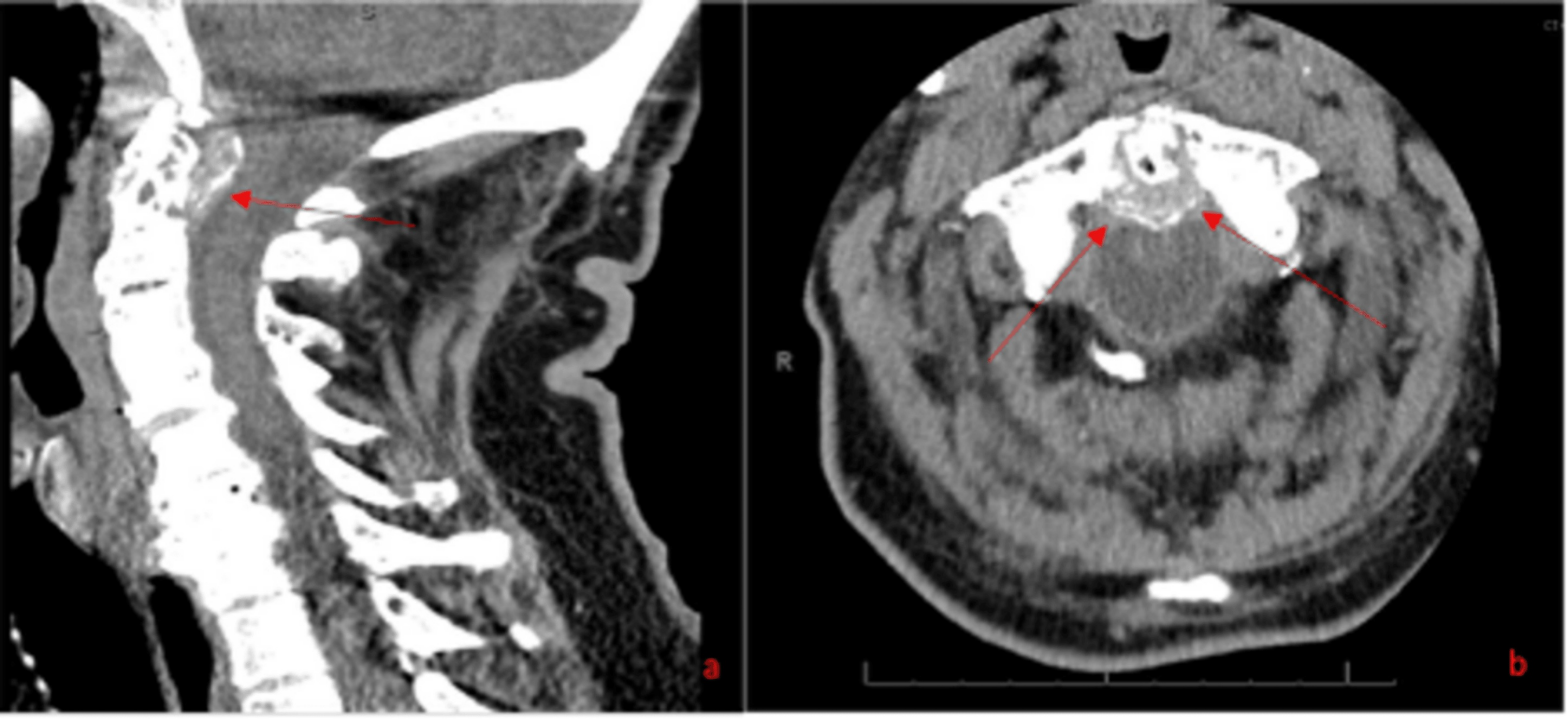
Sagittal (a) and axial at the level of C1 (b) views of the cervical spine on CT scan without contrast. Red arrows point to the crowned dens in the different views. Also visualized are the extensive erosive changes involving the odontoid process and base of the dens.
